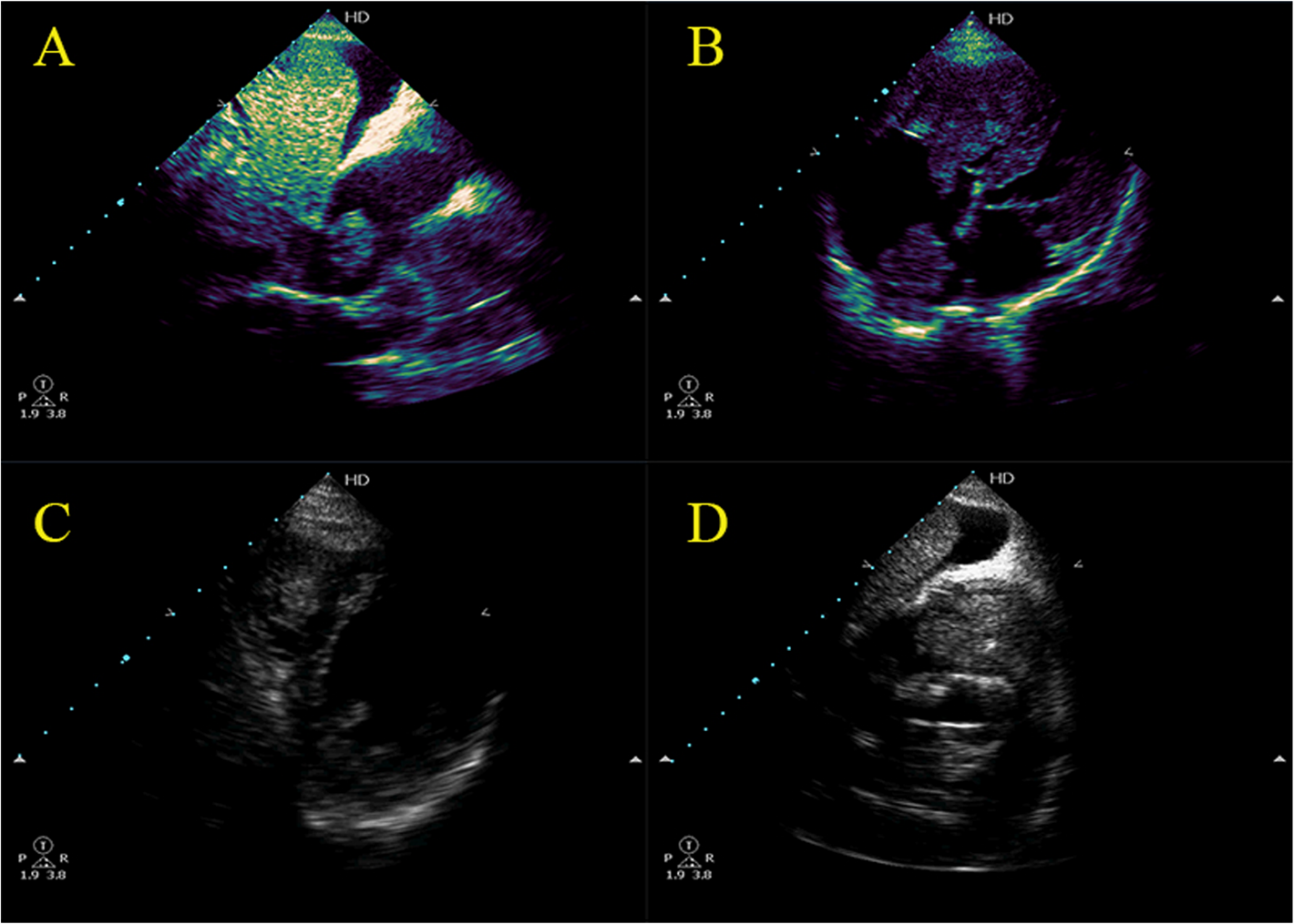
a–d Transthoracic Echocardiography revealing a large solid mass extending through IVC to RA with another highly mobile cauliflower mass at the RV apex occupying The RV cavity, protruding into RA through TV and nearly obliterating RVOT into the pulmonary artery
